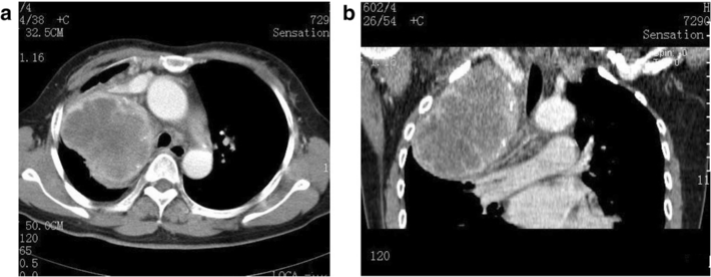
a Axial and (b) coronal contrast-enhanced CT images show a huge well-delineated shallowly-lobulated heterogeneously enhanced soft tissue mass in the right superior middle mediastinum with irregular hypodense areas of necrosis and multiple speckle-strip calcifications
